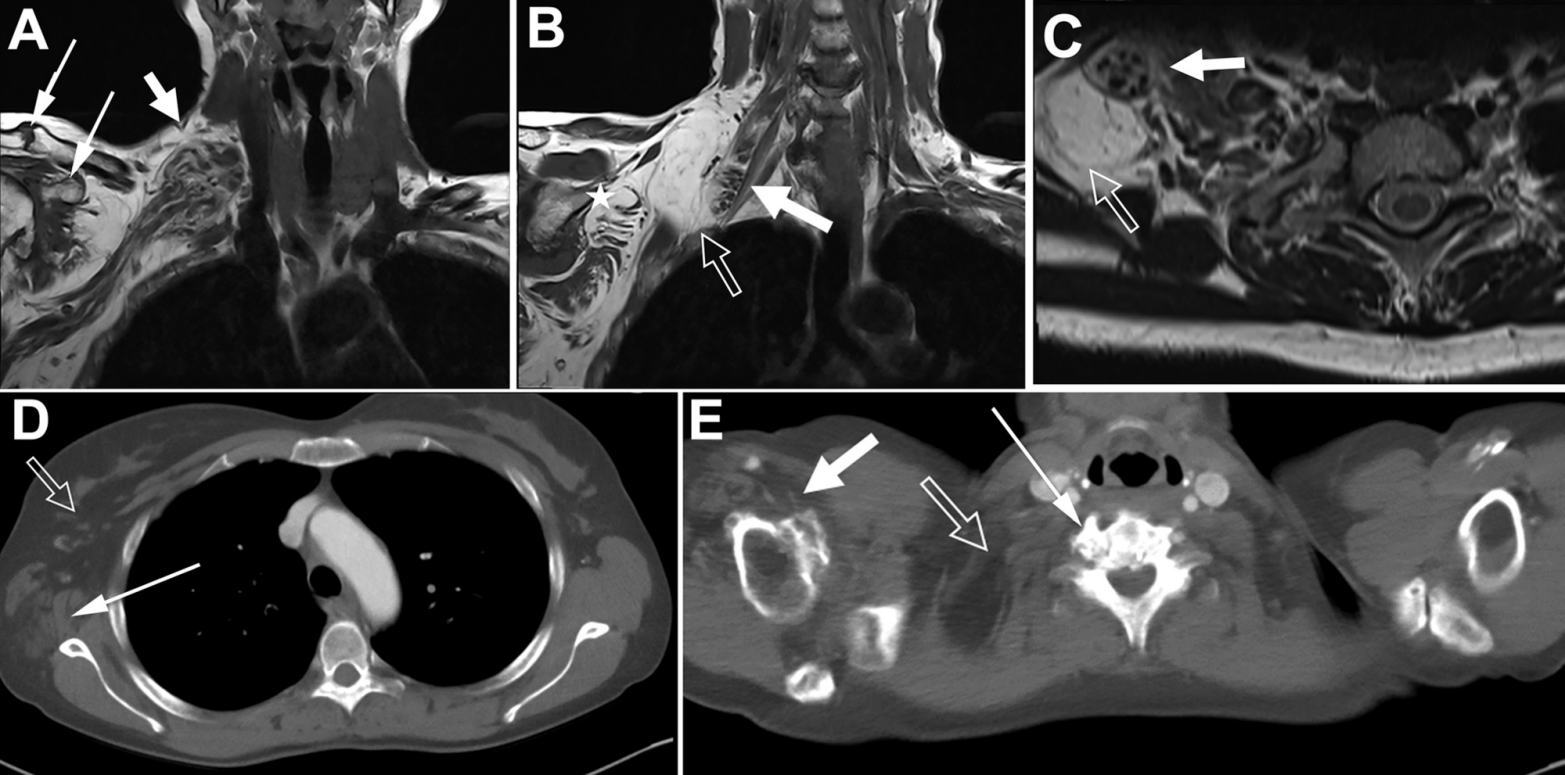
MRI and CT images of the right brachial plexus before exploration. (A) The T2-weighted coronal image demonstrates the enlargement of the entire brachial plexus (thick arrow), as well as overgrowth of the acromion and coracoid process (thin arrows); (B) An extraneural lipoma (hollow arrow) was posterior to the affected nerves (solid arrow) after they exited the scalene triangle. The nerves of the brachial plexus demonstrate the classic cable-like LN appearance involving the upper, middle, and lower trunks. Fatty infiltration of the subscapularis muscle is visible (star); (C) The same relationship can be seen in the axial T2-weighted cross-sectional imaging depicting the cable-like appearance of the brachial plexus (solid arrow) and the extraneural lipoma (hollow arrow); D & E: Computed tomography images of the chest before brachial plexus exploration; (D) Axial image at the arch of the aorta demonstrates asymmetric subcutaneous adipose (hollow arrow) and fatty infiltration of the latissimus dorsi muscle (thin arrow); (E) Axial image at the level of C7-T1 demonstrates marked lateral neuroforaminal osteophyte formation (thin arrow), bulky hypertrophy of the brachial plexus with associated lipoma (hollow arrow), and osteophyte formation of the right humerus (solid arrow) in sharp comparison to the normal left side.
